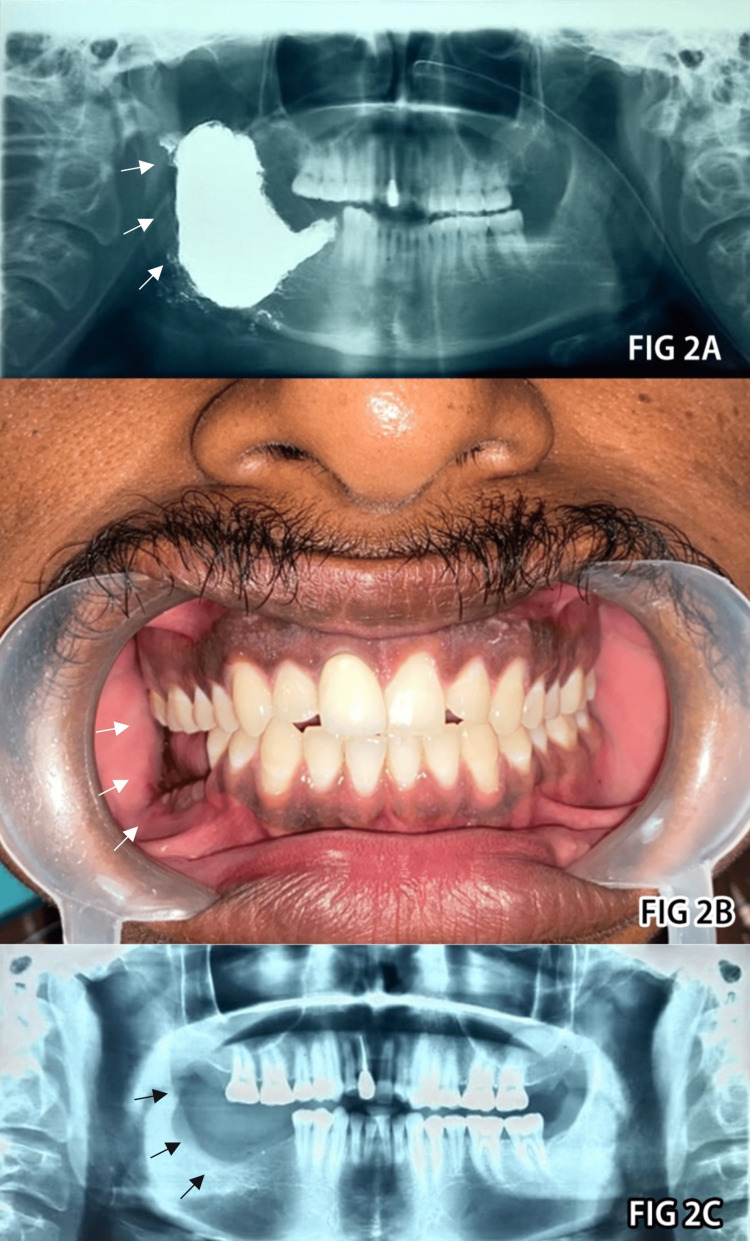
Postoperative radiographic and clinical presentation. A: A radiolucent area of filled iodoform gauze in immediate postoperative panoramic. B and C: The clinical and radiographic healing areas.
